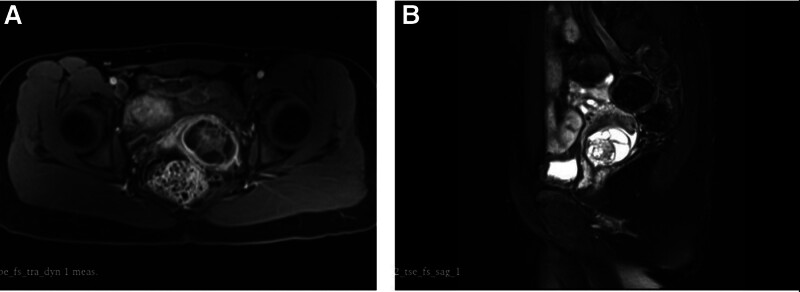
MRI images of patient 1. MRI = magnetic resonance imaging.
